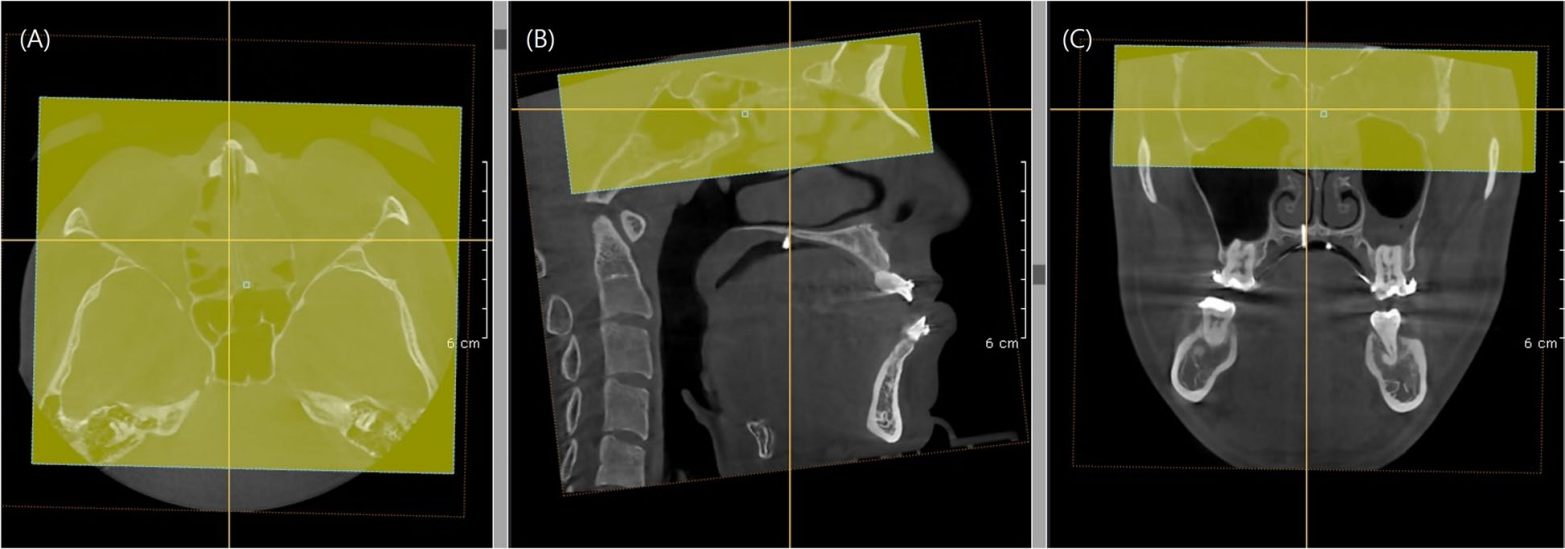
Superimposition of 3D-CBCT images with cranial base structures using the OnDemand3D software (CyberMed Inc., Seoul, Korea). The boxes indicate the areas of superimposition. (A): axial view; (B): sagittal view; (C): coronal view
